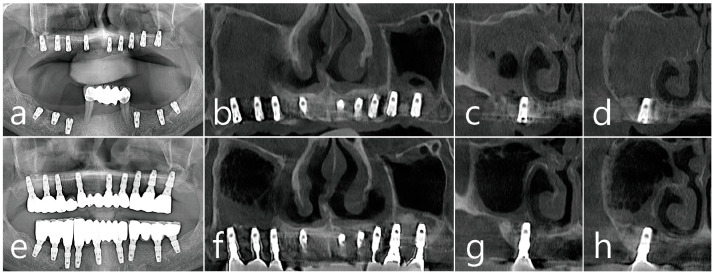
Case 1. Postoperative radiographs. (a) Panoramic radiograph of the right maxillary posterior area after surgical drainage, sinus floor augmentation, and implant placement. Panoramic (b) and coronal (c,d) images of CBCT obtained immediately after the surgery. Nasal polyps were noted. (e) Panoramic radiograph after 1-year of loading. No crestal bone loss was observed. Panoramic (f) and coronal (g,h) images of CBCT after 1-year of loading. Limited mucosal thickening lining the augmented sinus floor was shown. Patency of the natural ostium without nasal polyp was shown. CBCT; cone beam computed tomography.
